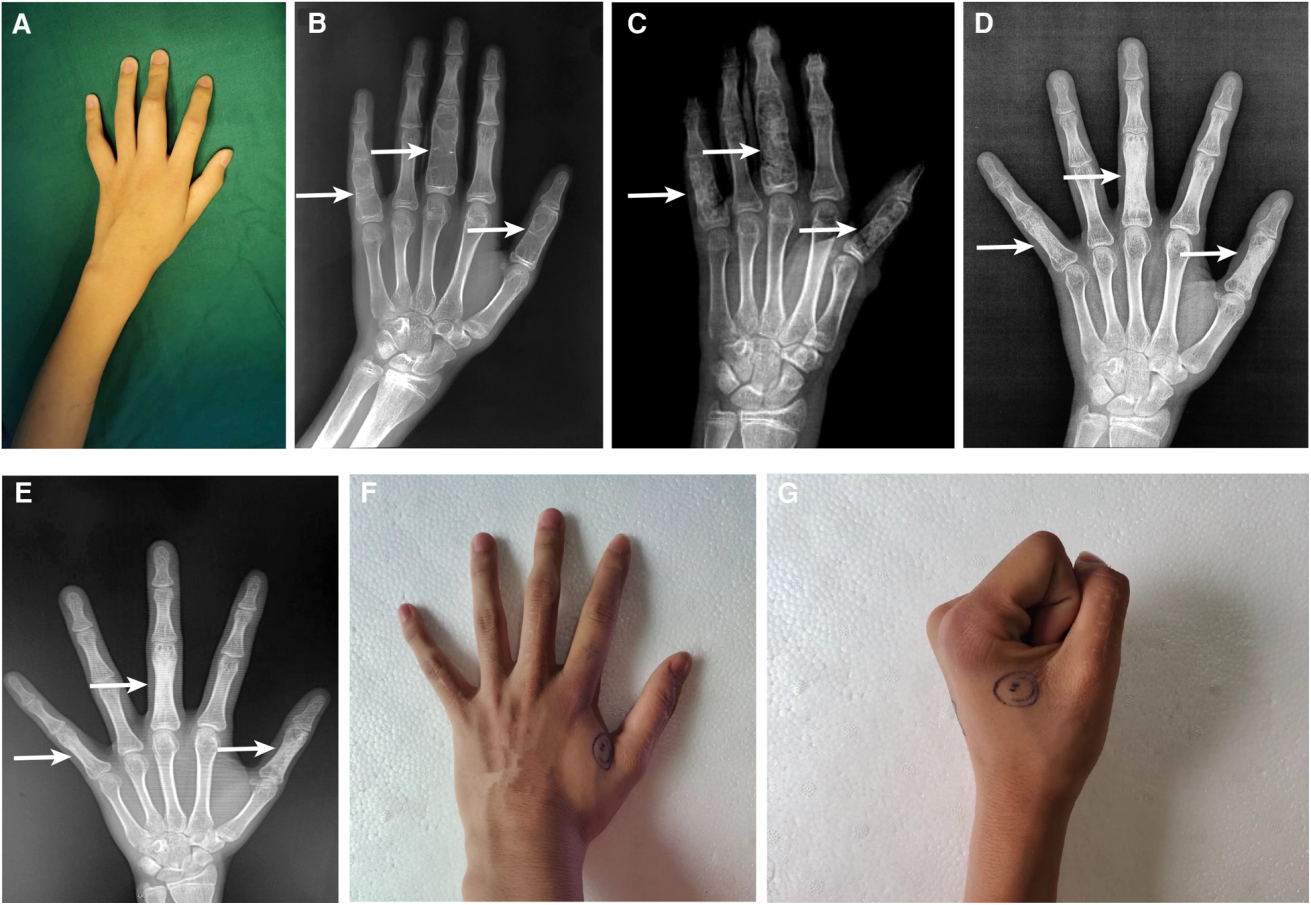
Clinical and radiographical results before and after surgery on the proximal phalangeal bone of left thumb, middle finger, and little finger. The fusiform eminence could be seen at the proximal phalangeal bone preoperatively (A). The preoperative x-ray (B); 2 days postoperatively (C); 7 months postoperatively (D); and 18 months postoperatively (E); white arrow indicates the locations of intraosseous hemophilic pseudotumor. The appearance of proximal phalanges (F) and the range of motion of metacarpophalangeal joints (G).
